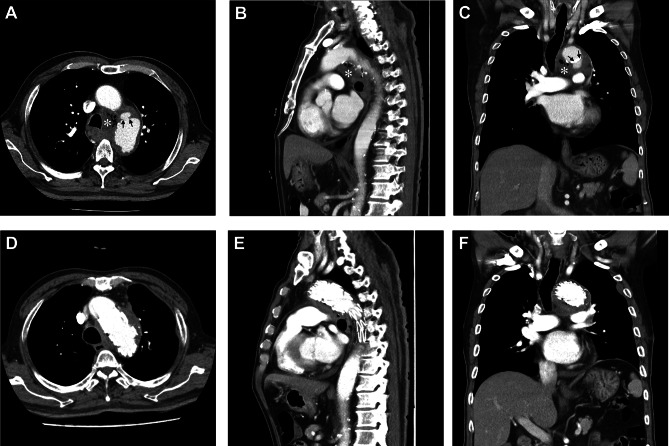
Axial, sagittal, and coronal views of contrast-enhanced chest computed tomography at admission (A-C) and on hospital day 49 (D-F). (A-C) Mediastinal fluid collection with mottled air density in the left lower paratracheal region (*asterisk*) and focal rupture in the distal portion of the aortic arch (*arrows*). (D-F) Improvement of the mediastinal abscess with favorable stent patency
